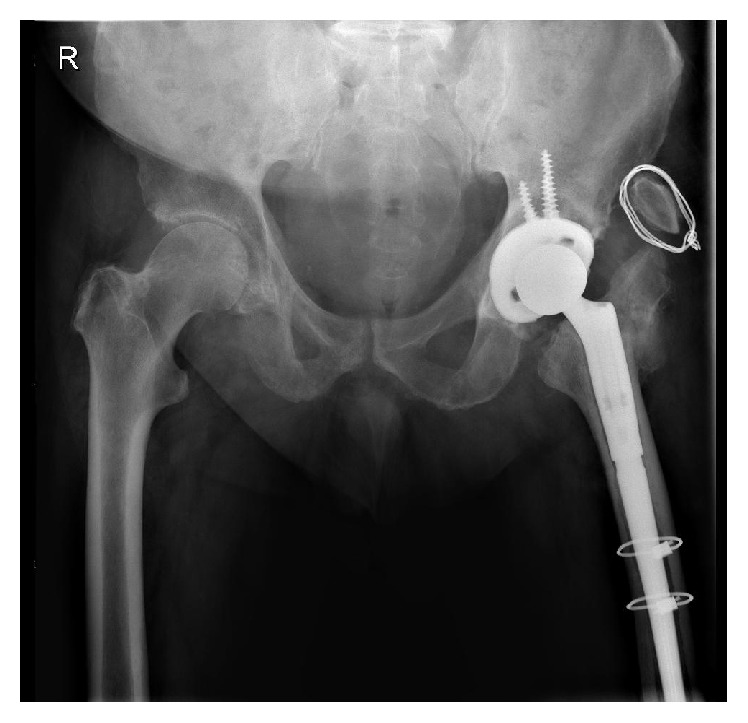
Postoperative anteroposterior pelvic plain film radiographs showing second-stage THR revisions in situ.
